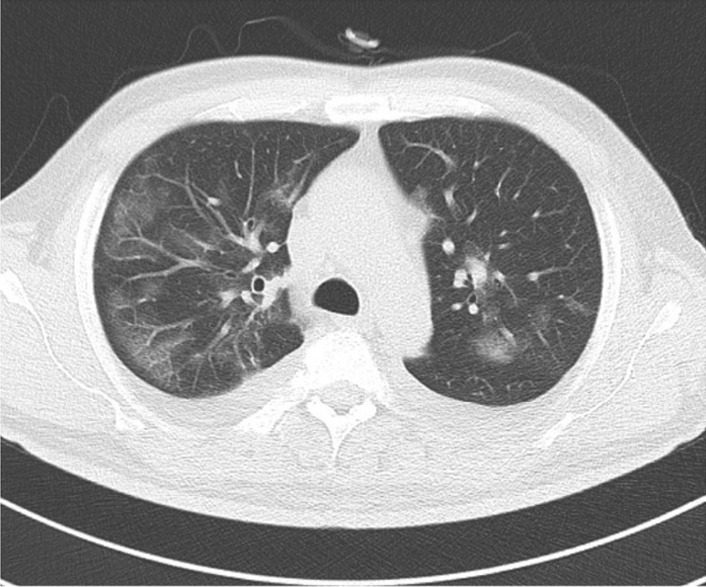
Chest CT image of pneumonia. (Case 7)
